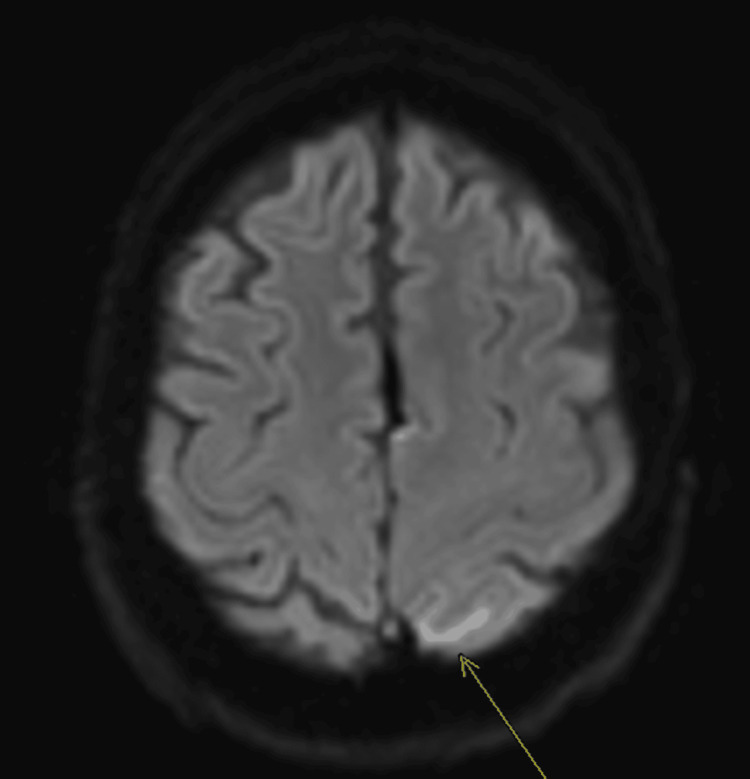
Axial T2 FLAIR MRI showing patchy areas of pial hyperintensity in the left subparietal region (arrow). These findings correlate anatomically with the angiographic 'tumor blush' seen in Figure 2, confirming leptomeningeal metastatic involvement. FLAIR: fluid attenuated inversion recovery
